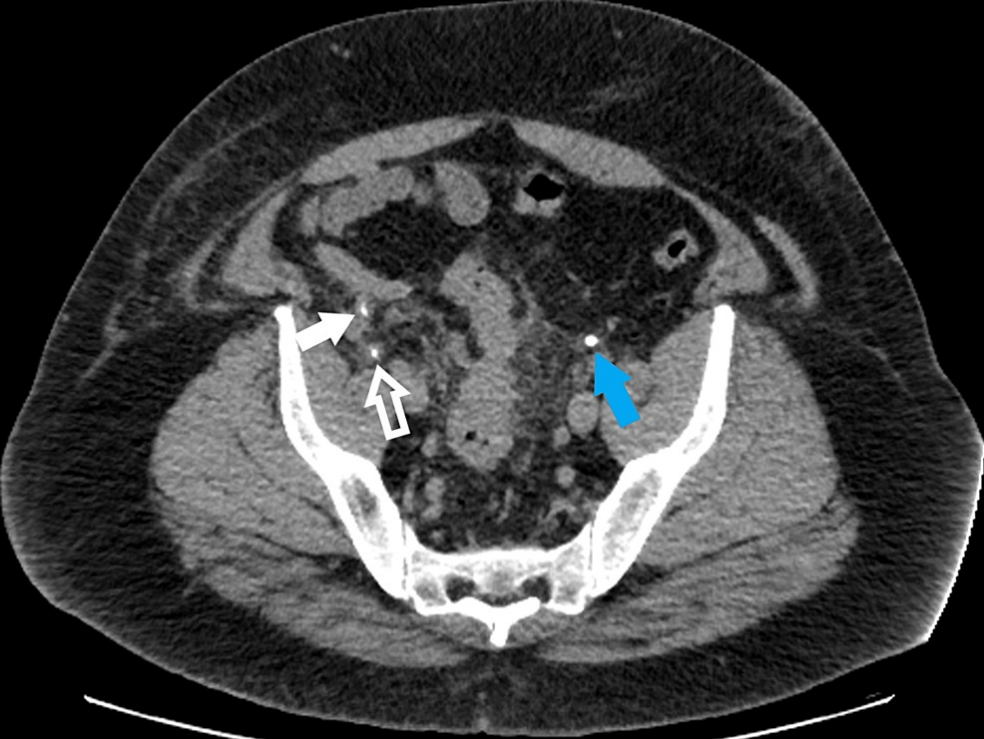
Post-operative CT Intravenous Pyelogram showing left ureter (blue arrow), proximal right ureter (solid white arrow) and distal right ureter (hollow white arrow).
